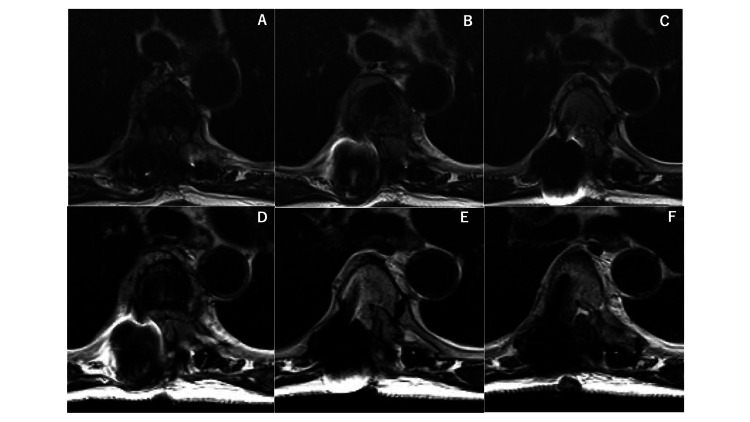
Magnetic resonance images before and after the second surgery MRI T2-weighted images show the recompression of the spinal cord by the tumor (A), leading to a second operation (B).
